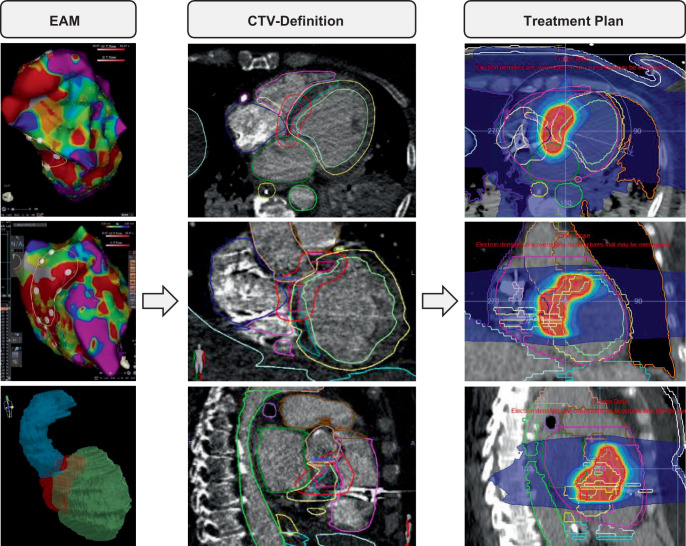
From the left*: EAM of the left ventricle (superior–inferior and anterior–posterior views, 3D reconstruction; voltage range 0.2–0.8 mV). Defined STAR target (CTV, *pink*; PTV, *red*) after transfer from EAM to the planning CT (transversal, coronal, and sagittal views). Radiotherapy plan dose distribution with a single dose of 25 Gy. *EAM* electroanatomical mapping, *CTV* clinical target volume
